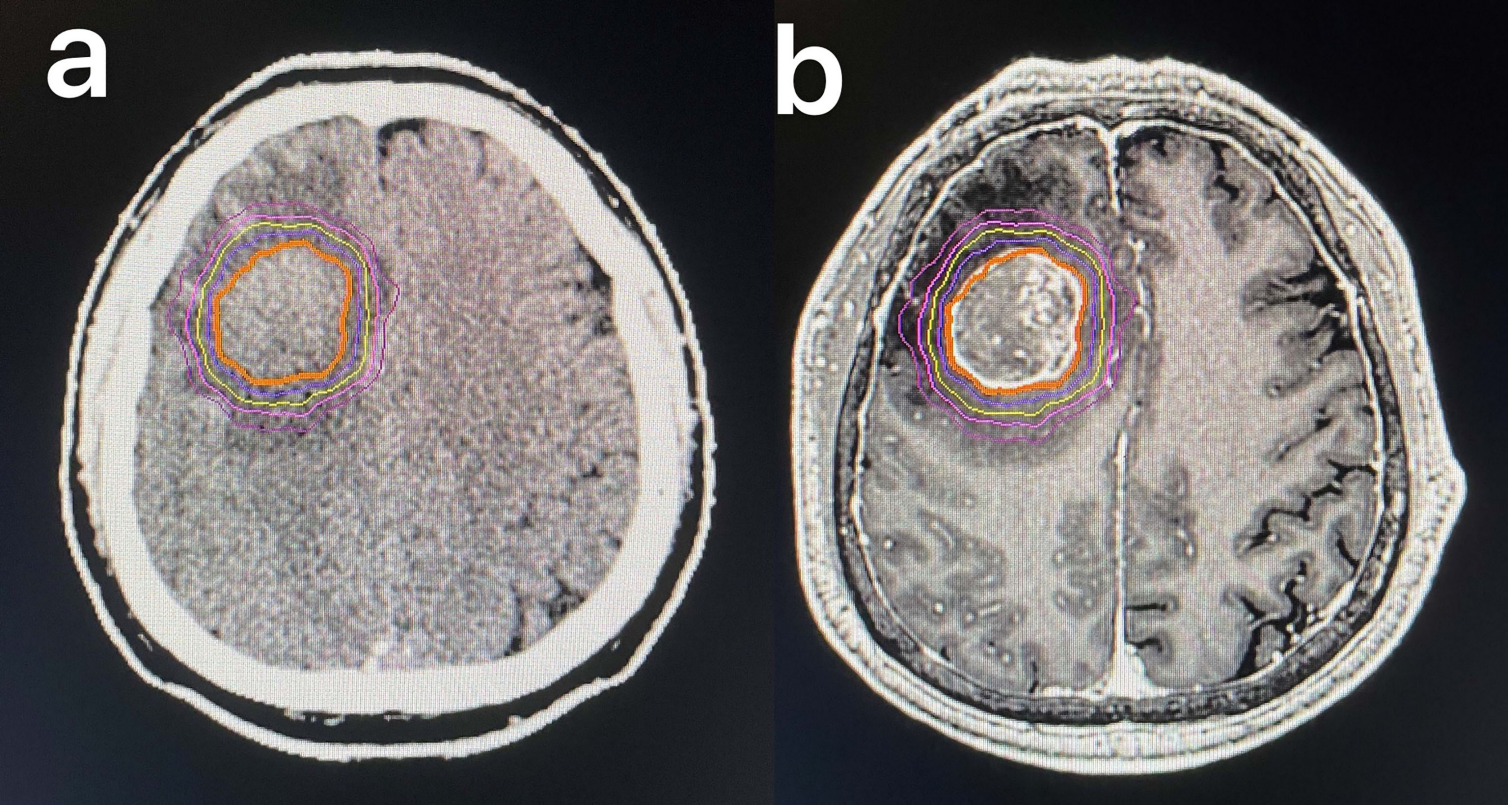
SBRT target area in a 58-year-old man with squamous cell lung cancer of the right frontal lobe: CT (A) and MRI (B). SBRT, stereotactic body radiotherapy.
